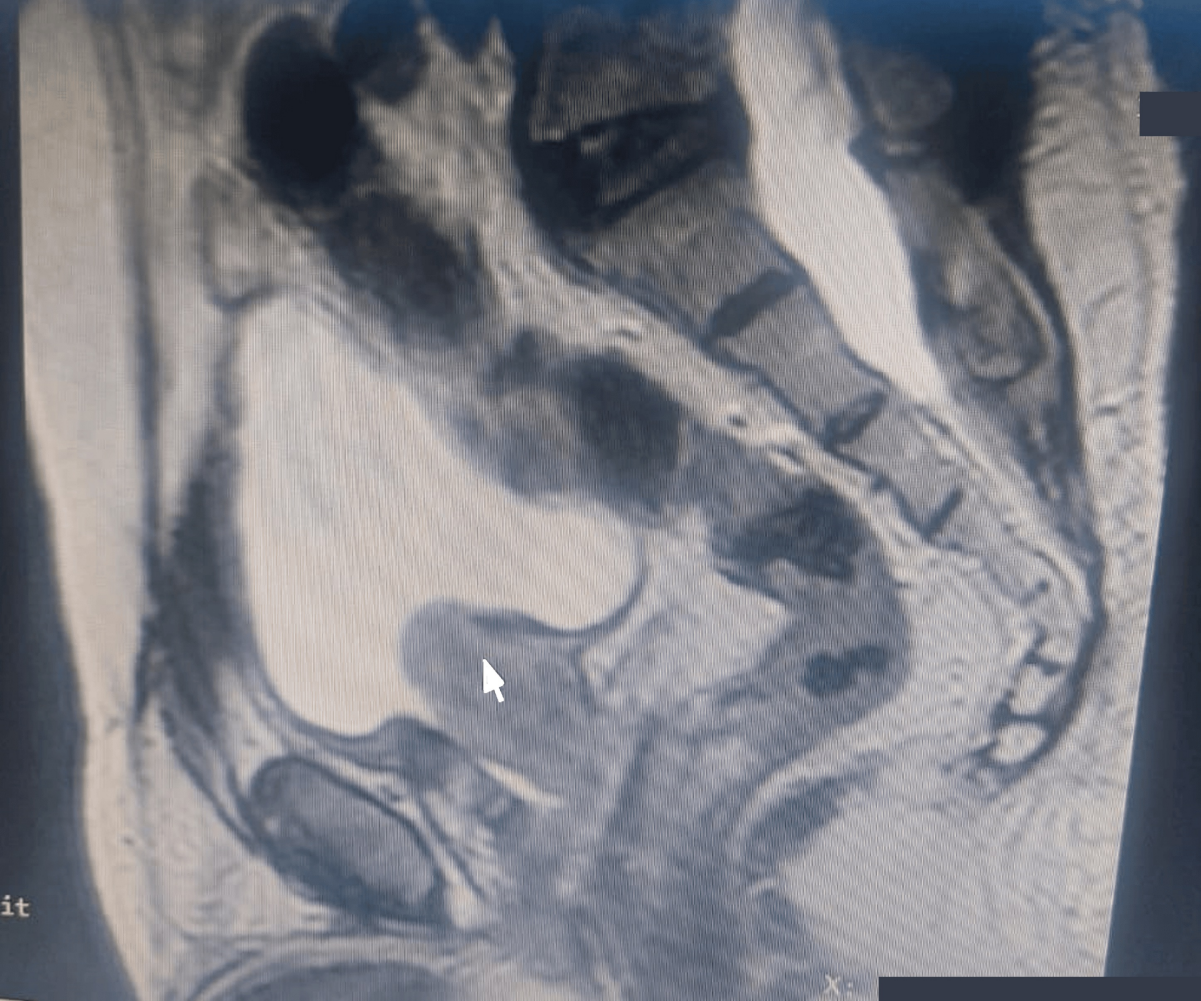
Pelvic MRI showing enlarged prostate (white arrow)
